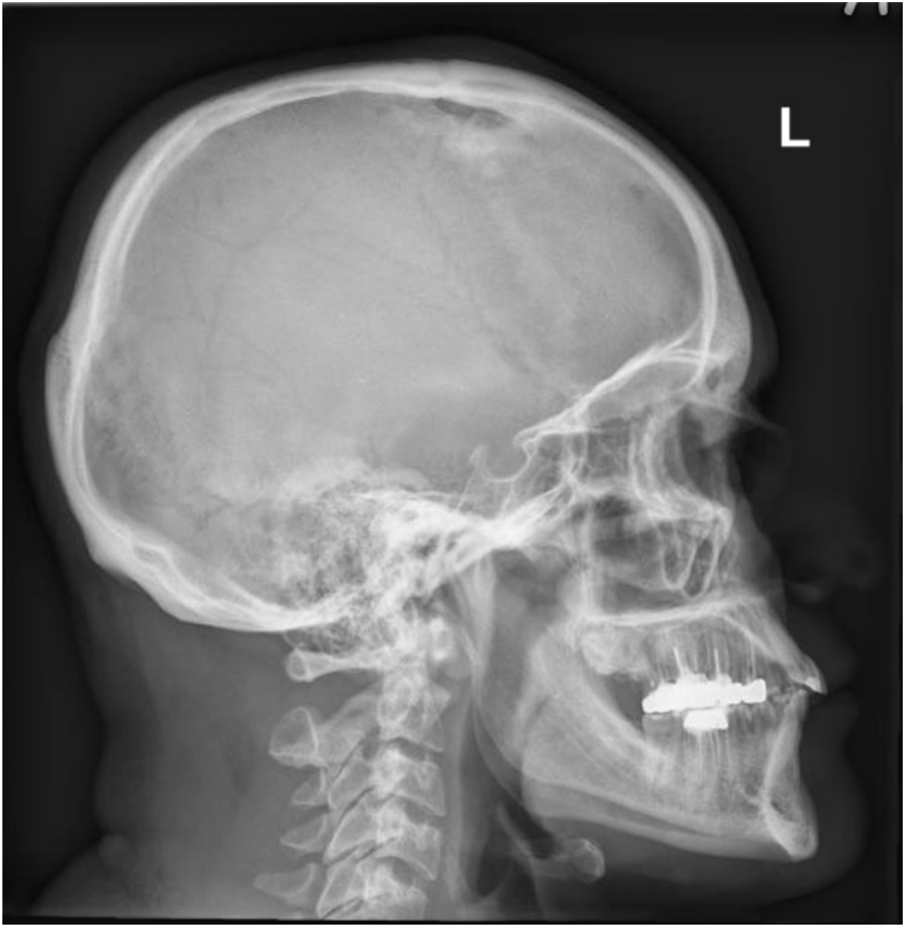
Skull x-ray showing osteolytic lesion.
